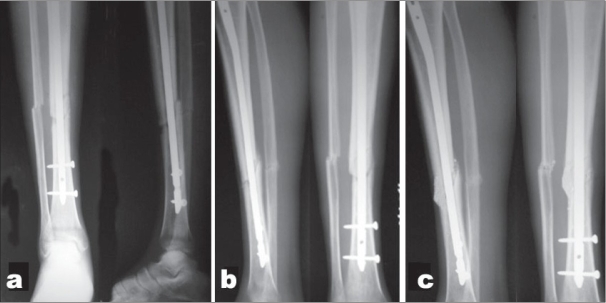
(a) Postoperative radiograph AP and lateral of the fracture showing good reduction and fixation with unreamed tibial nail (UTN) and double distal locking in situ. (b) Radiograph lateral and AP after 12 weeks of surgery, showing ongoing bridging of fracture site and consolidation. (c) Radiograph lateral and AP after 24 weeks of surgery, in which healing is almost complete.
